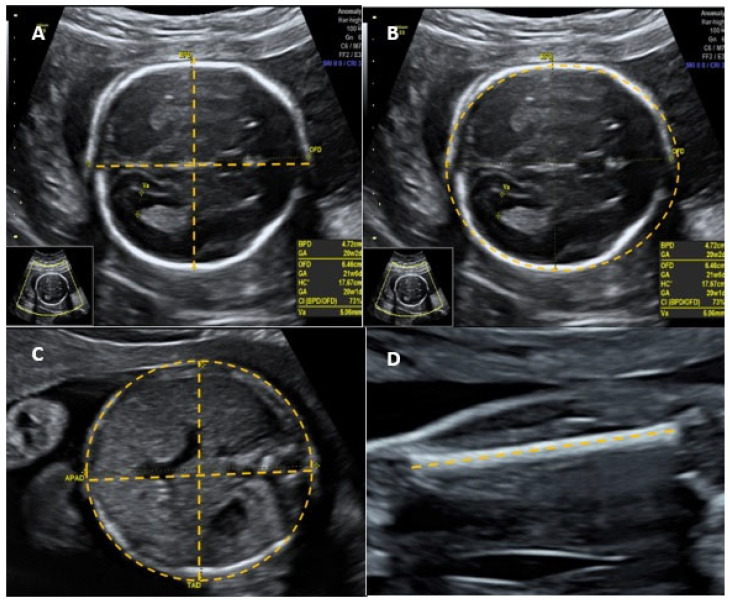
Measurements of biparietal diameter (BPD) (A), head circumference (HC) (B), abdominal circumference (AC) (C), and femur length (FL) (D) according to the International Society of Ultrasound in Obstetrics and Gynecology (ISUOG) guidelines.
